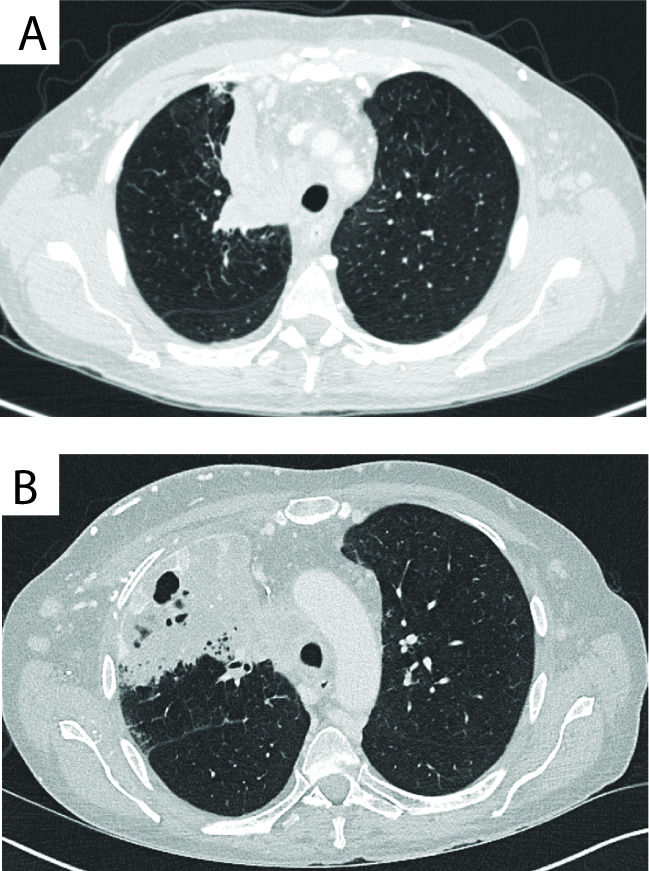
Development of pulmonary TB on triplet chemotherapy. Comparison of diagnostic CT scan performed 2 weeks before treatment (A) with the CT scan at the time of TB diagnosis (B) shows regression of the tumour with immune checkpoint inhibition but new consolidation with cavitation. Adapted from Crawley et al.^56^
